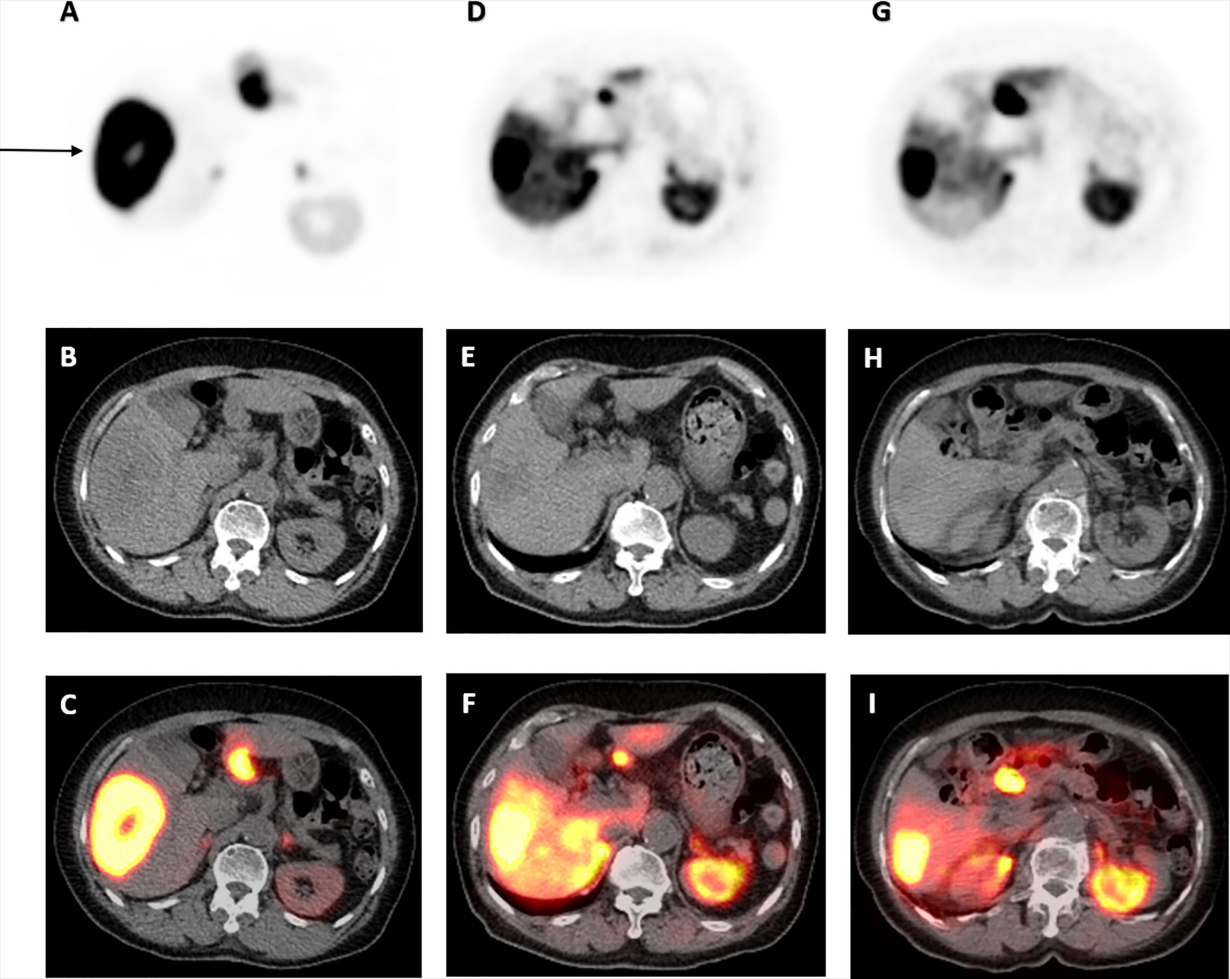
68Ga-DOTATATE PET/CT scan images of dominant right liver lobe lesion pre- and post-177 Lu-DOTATATE. Axial view images of ^68^Ga-DOTATATE PET/CT scan demonstrating interval reduction in size in dominant right liver lobe lesion (arrow) on PET, low-dose CT and PET/CT fusion (top to bottom) from baseline, to 1 and 4 years after ^177^Lu-DOTATATE therapy (left to right). Physiological uptake in the spleen and remainder of the liver is also visualized.
